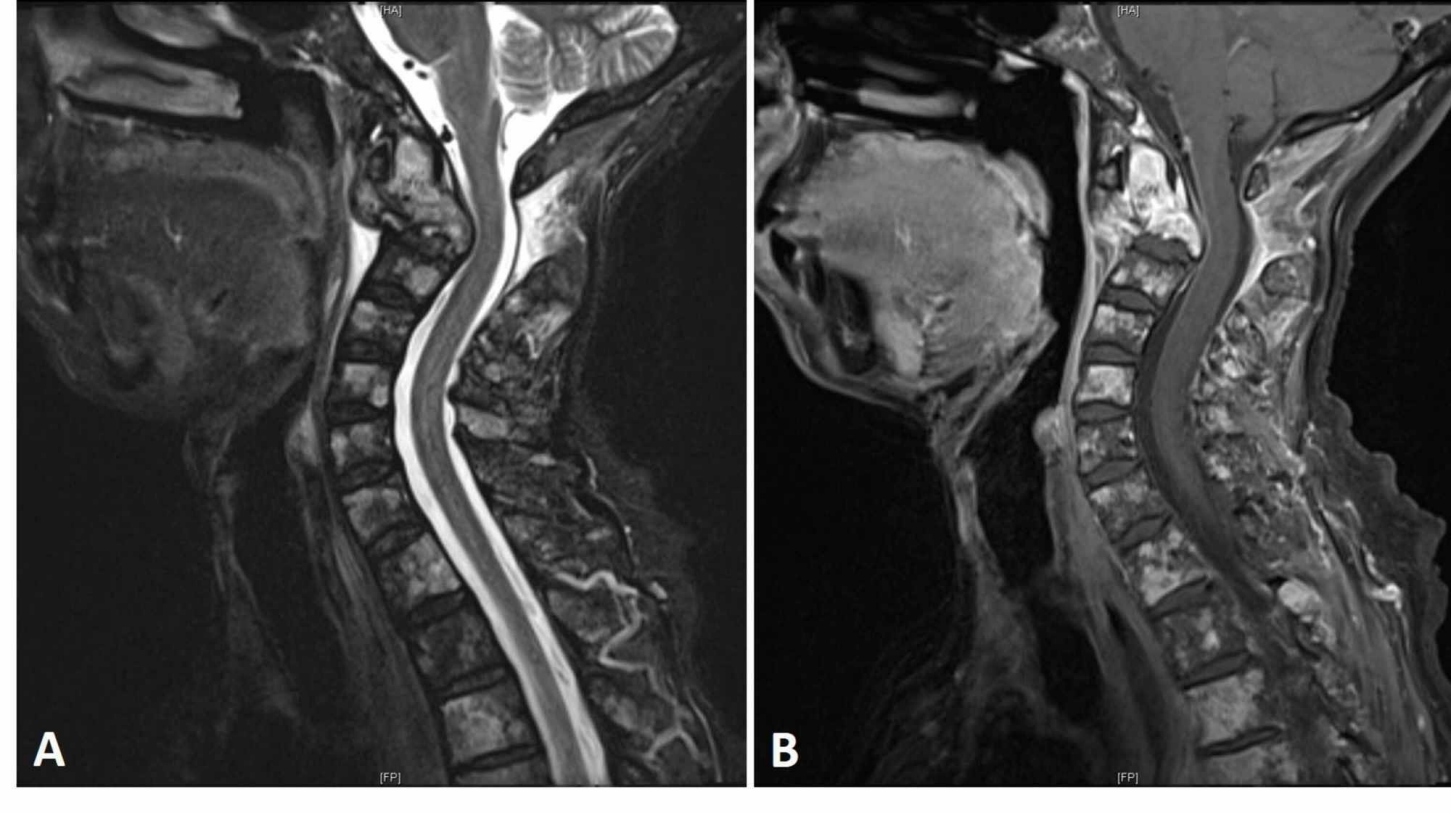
Preoperative MRI of the cervical spine with contrast MRI of the cervical spine with contrast showing extensive osseous metastatic disease and an upper cervical spinal kyphotic deformity due to the C2 pathological fracture (A: sagittal T2, and B: sagittal T1 postcontrast).
